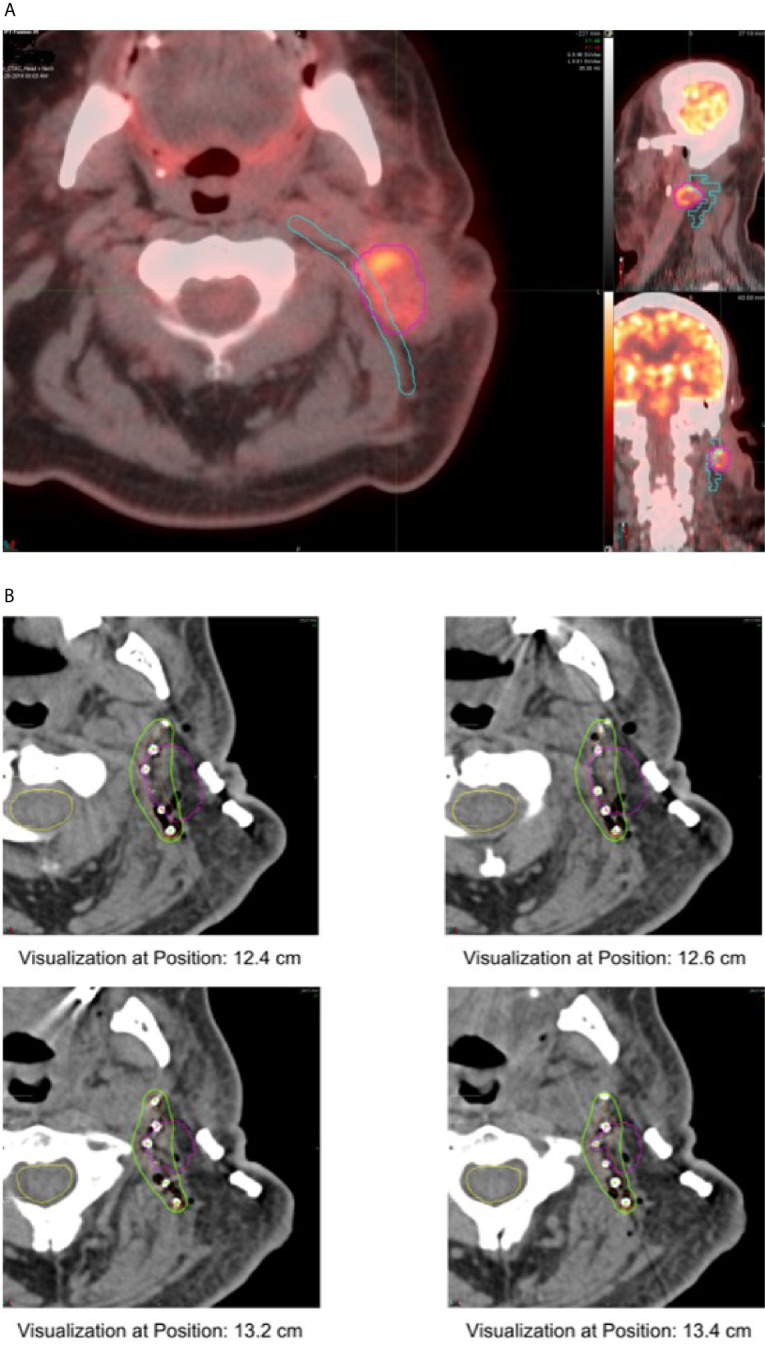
(A) Pre-operative plan showing Cs-131 strand implant location along predicted tumor bed. (B) Post-operative plan showing implanted Cs-131 seeds on diagnostic CT, pre-operative tumor contour in purple.
